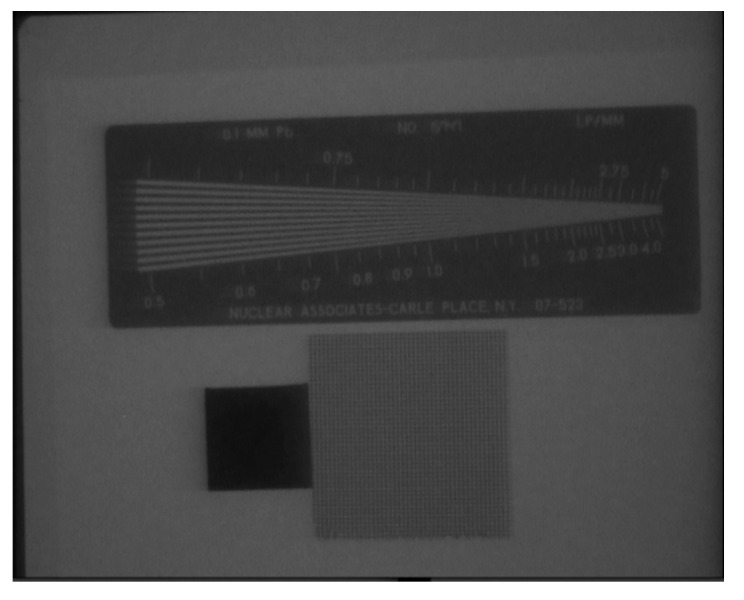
Resolution test of X-ray dectetor. 1.2 lp/mm is observed.
